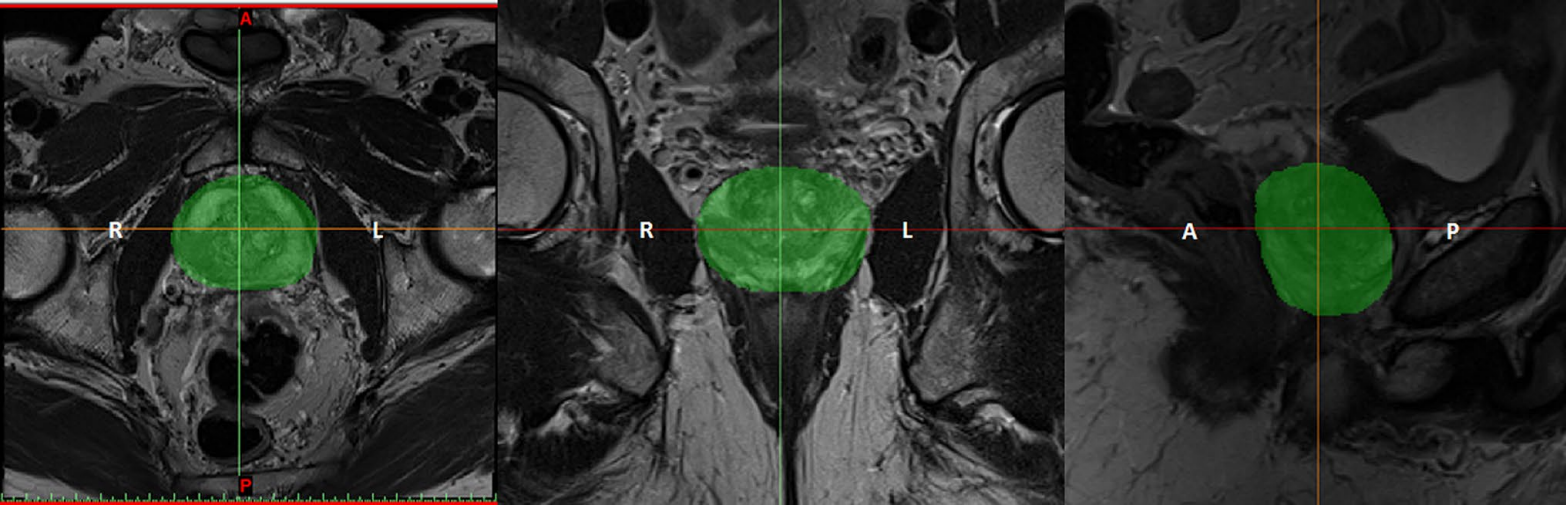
Segmentation of the prostate (*green* area) in three different views of MR imaging, from *left* to *right* axial, coronal and sagittal
